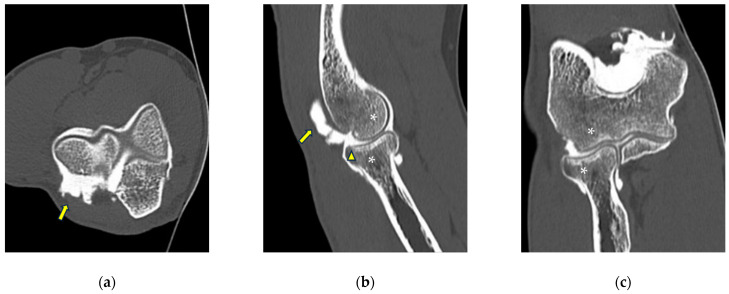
CT-arthrography of a professional motocross rider with chronic elbow pain due to repeated microtraumatism. (a,b) Axial and sagittal images show pathological widening of the postero-lateral recess (yellow arrows); (b,c) sagittal and coronal images show articular asymmetry of the humero-radial joint (asterisks), diffuse cartilage fraying and a full-thickness chondral defect (grade IV) of the posterior aspect of the radial head dish (yellow arrowhead).
